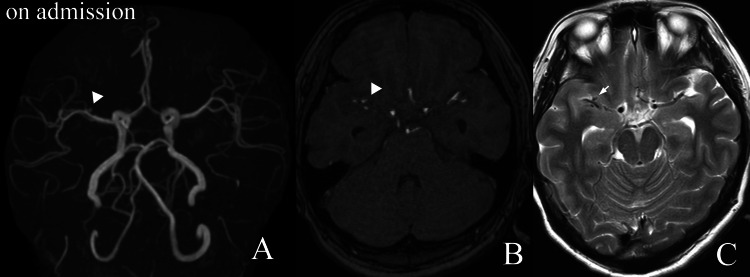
Magnetic resonance imaging findings at admission. (A) Time-of-flight magnetic resonance angiography (TOF-MRA) and (B) contrast-enhanced magnetic resonance angiography demonstrate a 2-mm saccular aneurysm arising from the M2 superior trunk of the right middle cerebral artery (arrowhead). (C) T2-weighted imaging shows no perianeurysmal signal change suggestive of thrombus formation (arrow).
